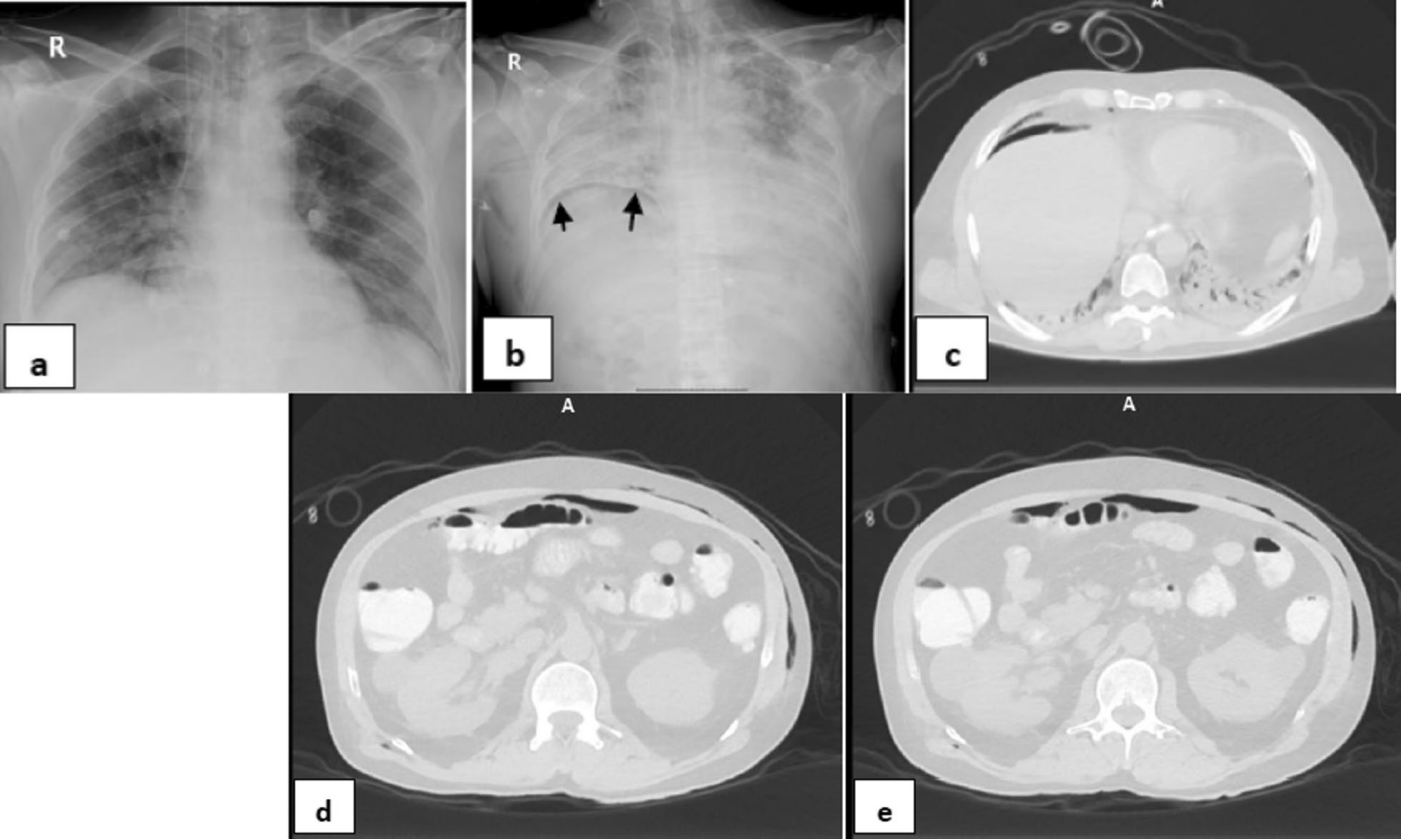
45-year-old male intubated 5 days after admission. a, b Two frontal chest radiographs show air under right diaphragmatic copula (black arrows). c–e series axial CT abdominal cuts demonstrate peumoperitonium and left lateral anterior abdominal wall surgical emphysema
